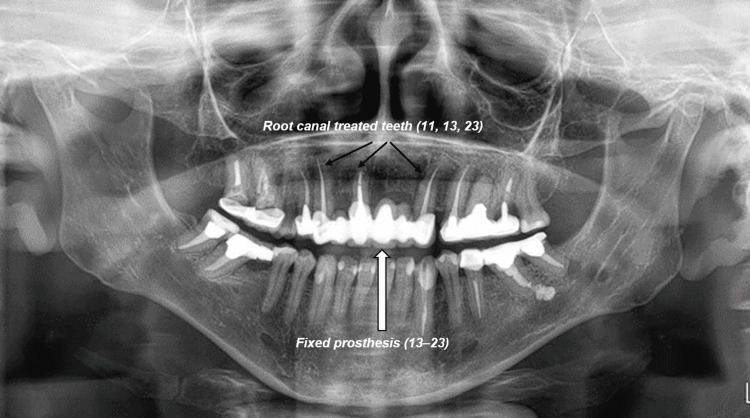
Panoramic radiograph at seven-year follow-up showing stable ceramo-metal bridge, intact alveolar bone levels, and no signs of periapical pathology or prosthetic failure
